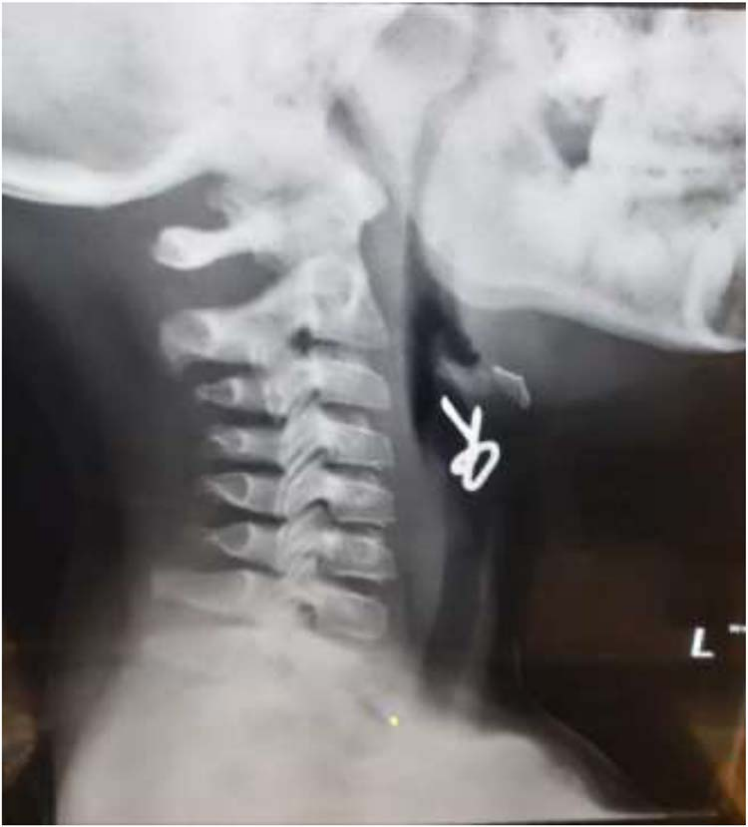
Lateral neck X-ray showing a radio-opaque object with sharp edges visible.
